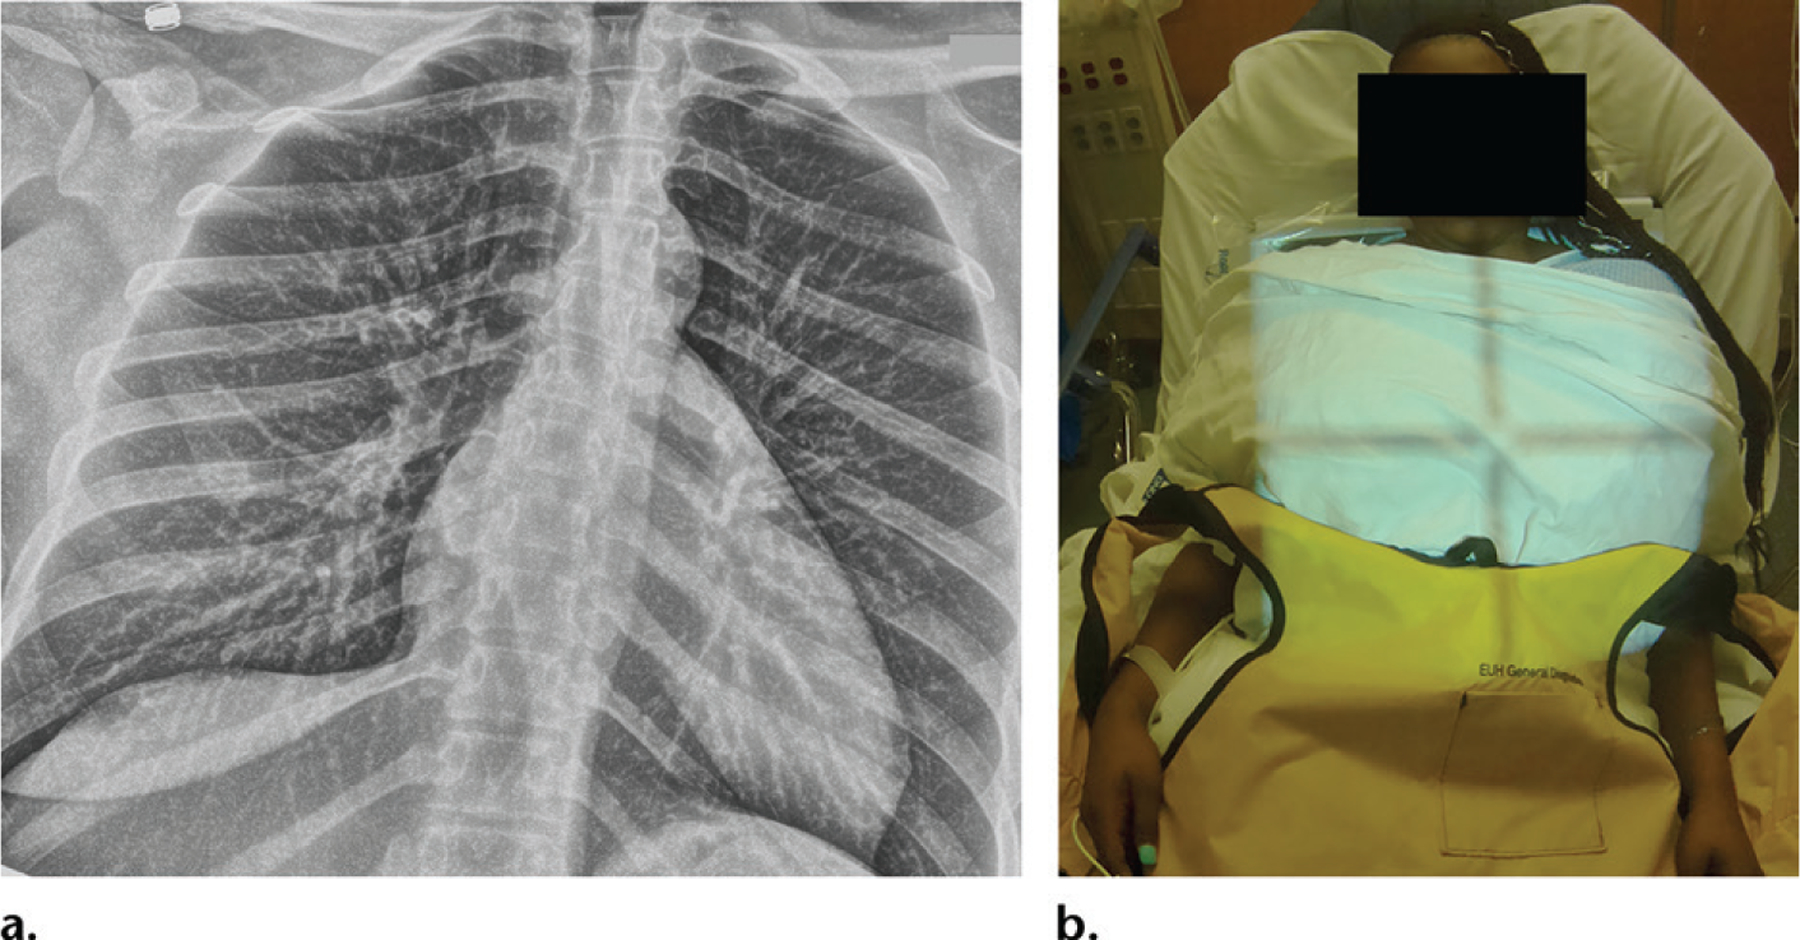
(a) Portable chest radiograph obtained in a young woman shows the lungs. (b) Photograph shows the patient with appropriate shielding of the lower abdomen and pelvis. Proper shielding is necessary to both reassure the patient and minimize radiation exposure. Documenting such maneuvers may become necessary from a medicolegal standpoint.
